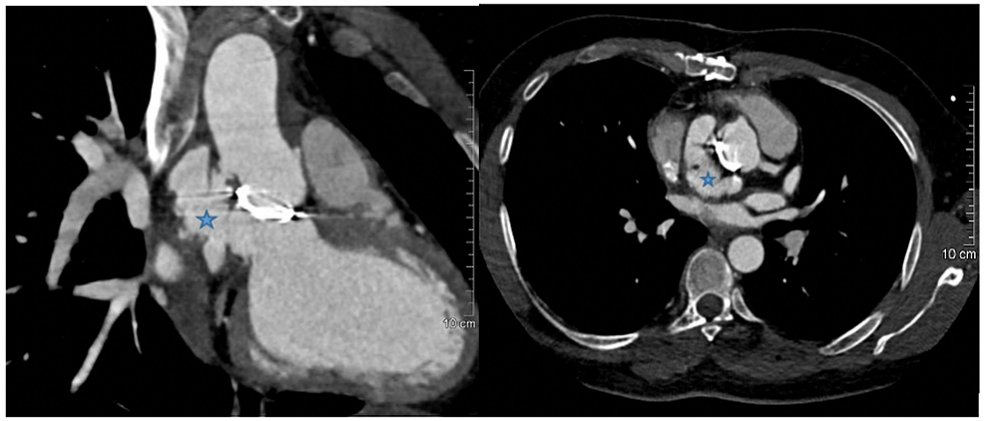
Cardiac computed tomography angiography (CTA) showing a lobulated pseudoaneurysm
